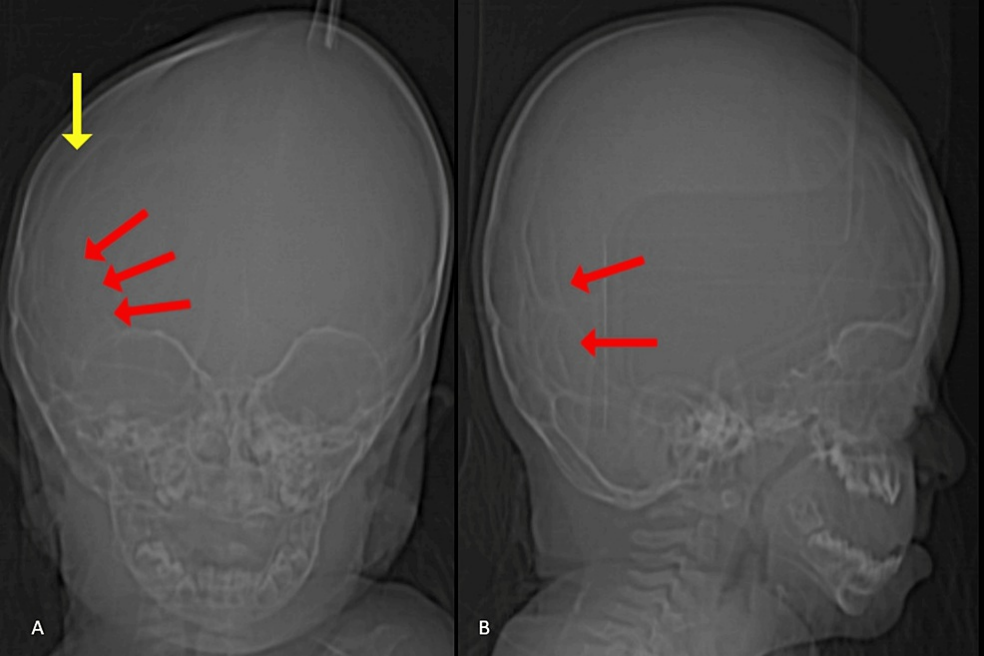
Anterior-posterior (A) and lateral (B) radiographs of the skull demonstrating copper-beaten appearance (red arrow) due to raised intracranial pressure and fusion of the right coronal sutures (yellow arrow).
